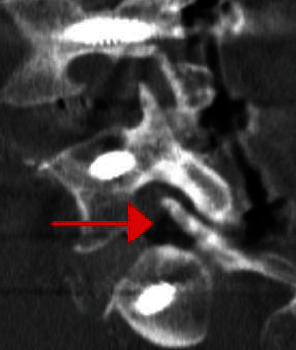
Postoperative sagittal CT scan demonstrating persistent L5 nerve root compression in the L5-S1 foramen (arrow).
